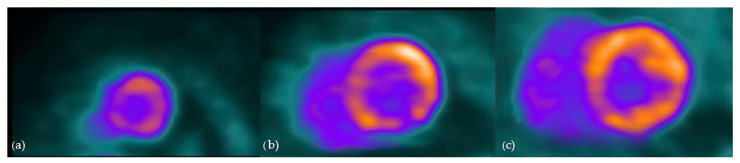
Cardiac PET in a patient with cardiac sarcoidosis: ^18^F-FDG uptake in most of left ventricle, consistent with active inflammation; No significant uptake in apex or mid-inferolateral segment, compatible with possible fibrosis ((a)—apex, (b)—mid-ventricle, (c)—basal).
